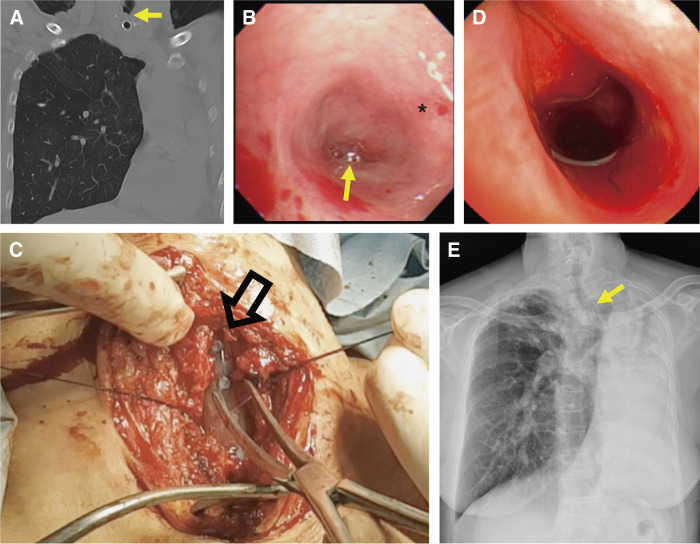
Perioperative imaging and bronchoscopic findings of refractory upper tracheal stenosis managed with open Dumon stenting. (A) Preoperative coronal chest computed tomography scan showing severe upper tracheal stenosis (arrow) above a Shiley tracheostomy tube, with a destroyed left lung. (B) Preoperative bronchoscopy demonstrating the cricoid cartilage (*) and pinhole upper tracheal stenosis (arrow). (C) Intraoperative image showing a longitudinal tracheotomy over the stenotic segment (hollow arrow) with a Dumon silicone stent positioned across the lesion. (D) Postoperative bronchoscopy showing the proximal stent margin at the level of the cricoid cartilage with a patent tracheal lumen. (E) Chest radiograph at the 6-year follow-up demonstrating the stent in situ (arrow) with maintained position and airway patency.
